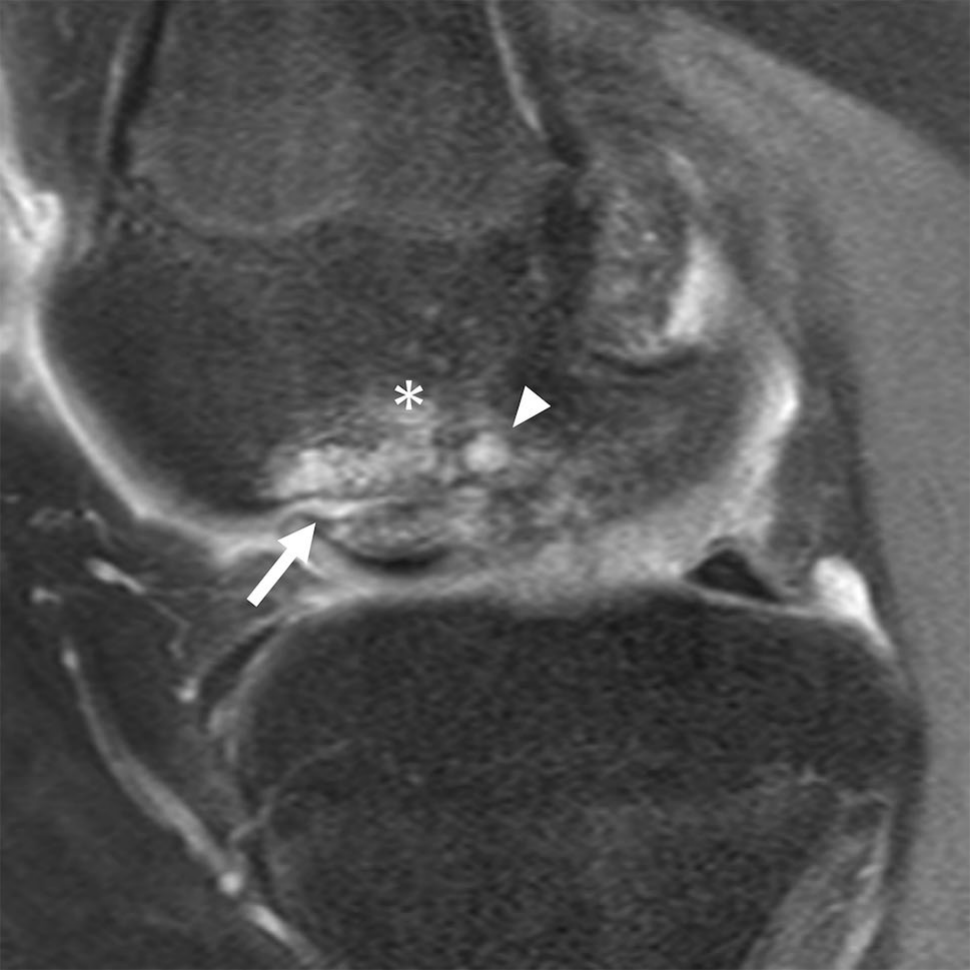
MRI of a 16-year-old boy with unstable OCD in the medial femoral condyle in the right knee. A sagittal FS-PDWI shows a rim of fluid signal intensity surrounding the OCD lesion (arrow). There is also cyst formation in the parent bone (arrowhead). Subtle bone marrow edema-like signal intensity can be observed around the cyst (asterisk)
